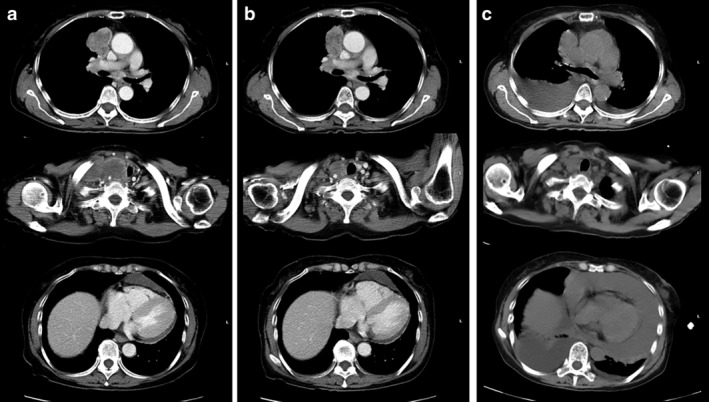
(a) A computed tomography (CT) scan of the chest showed a mass lesion in the right upper lobe. A right supraclavicular lymph node metastasis infiltrated into the right thyroid lobe before pembrolizumab treatment. Mild pericardial effusion was observed. (b) After three cycles of pembrolizumab therapy, CT scan showed a positive response in the primary lesion and lymph node metastasis. (c) A CT revealed a sudden increase in pericardial effusion and the tumor lesions were reduced after four cycles of pembrolizumab therapy.
